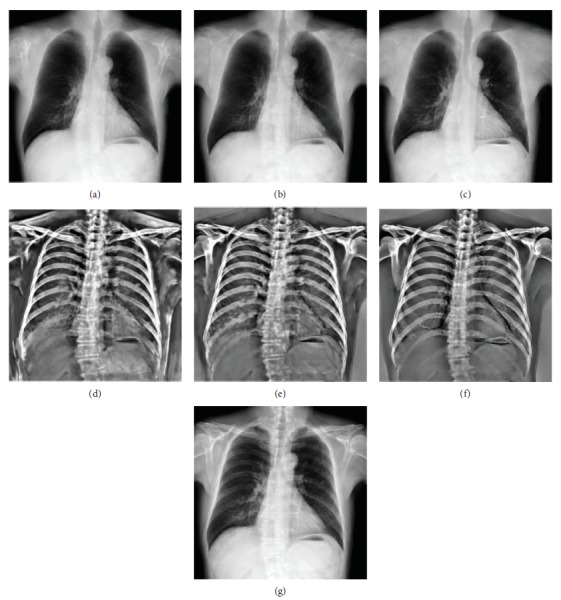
Comparison of the decomposition results through the MAP model and the locally weighted regression method: (a) the reconstructed soft tissue image through the locally weighted method; (b) the decomposed soft tissue image through the MAP model; (c) the DES soft tissue image of (g); (d) the reconstructed bone image through the locally weighted method; (e) the decomposed bone image through the MAP model; (f) the DES bone image of (g); (g) the standard chest radiograph.
